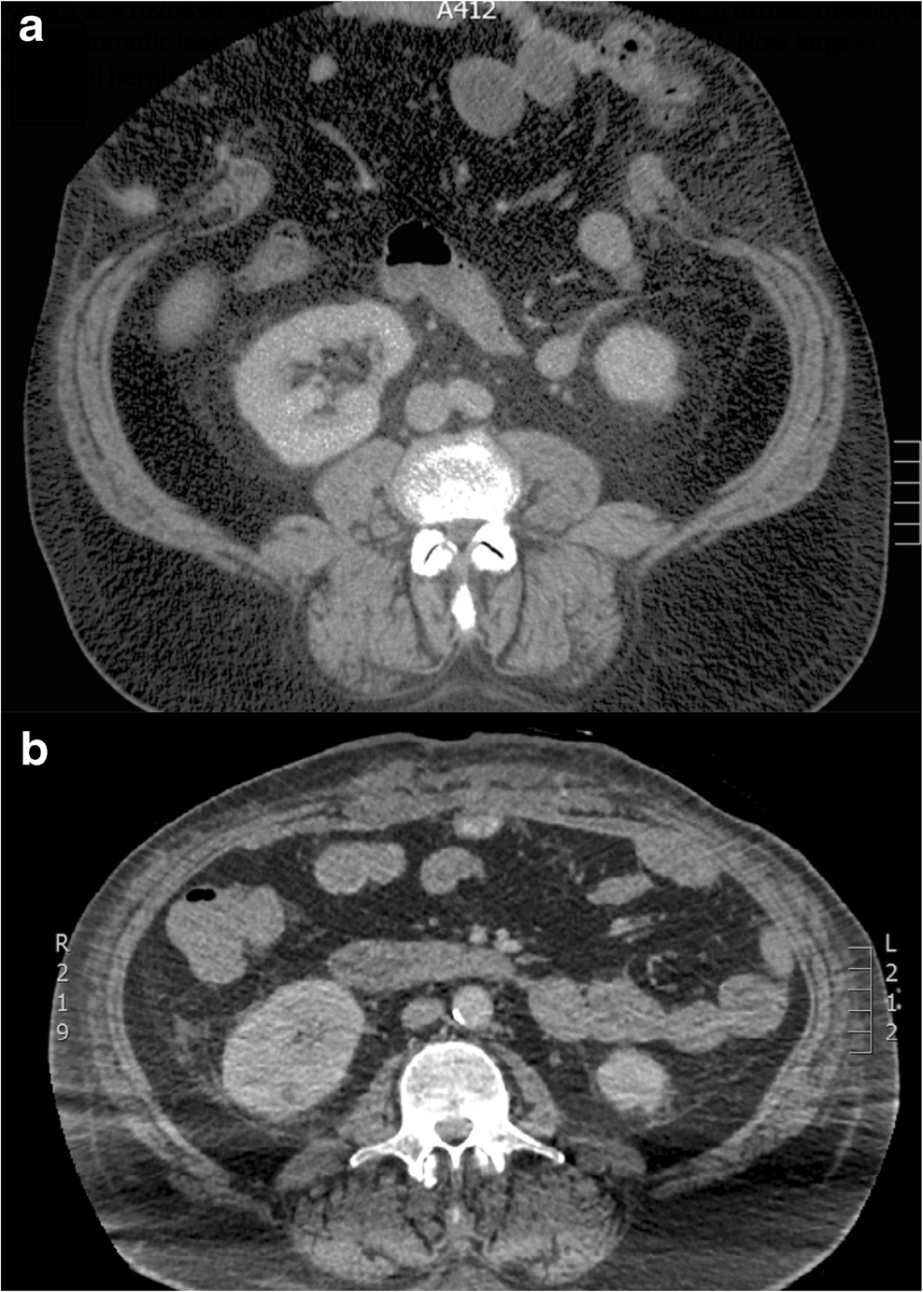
a Preoperative axial CT showing a huge ventral hernia following sigmoid colectomy. b Postoperative axial CT showing ventral hernia repair achieved by bilateral anterior component separation combined with an intraperitoneal mesh
